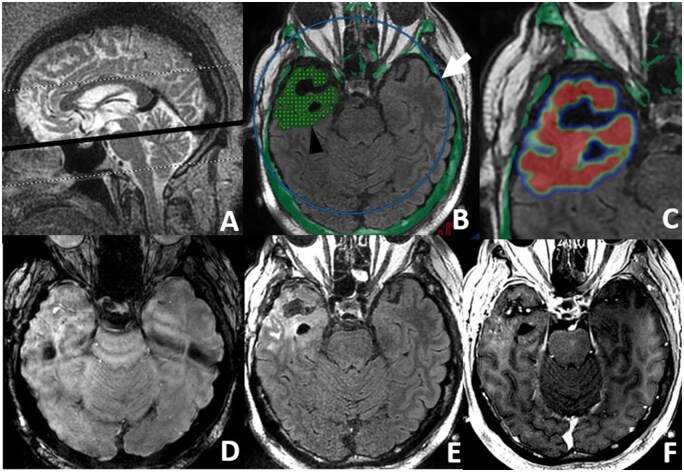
MRgFUS BBBO in a patient during the sixth cycle. “In-trasducer protocol.” (A) Pre-sonication T2w imaging, sagittal plane. The black line represents the reference slice for images B-F showing the predefined inclination maintained during the in-transducer protocol. (B) Targeting on pre-sonication FLAIR images. Two free-shape greeds of maximum 64 subspots covering target area (arrowhead). The treatment envelope, focused on tumoral location is also visible (white arrow). (C) Color-coded cavitation dose map. (D-F) Postprocedural in-trasducer SWAN, pc T2wFLAIR and T1w showing the effects of BBB opening.
